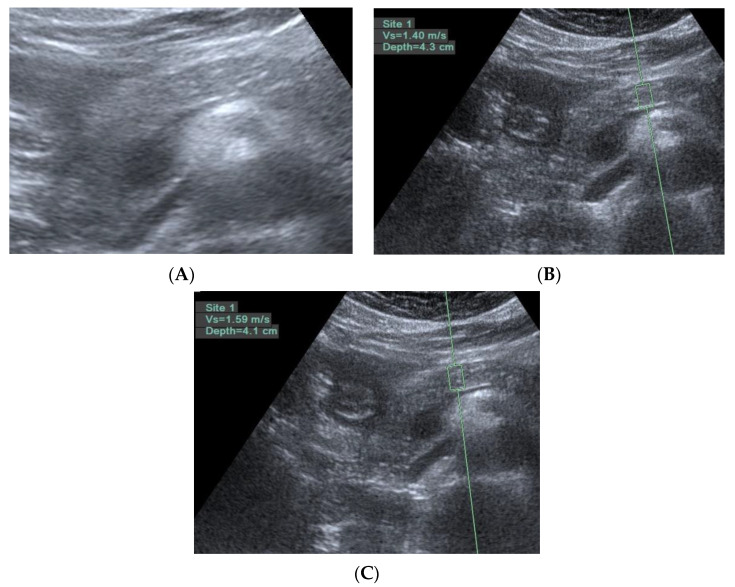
Three trans-abdomen sonographic images of the pancreas in the same patient. (A) Some hyperechoic foci in the pancreas; (B) if there are no hyperechoic foci in the region of interest, the pancreatic ARFI is 1.4 m/s; and (C) if there are hyperechoic foci in the region of interest, the pancreatic ARFI is higher (1.59 > 1.4 m/s).
